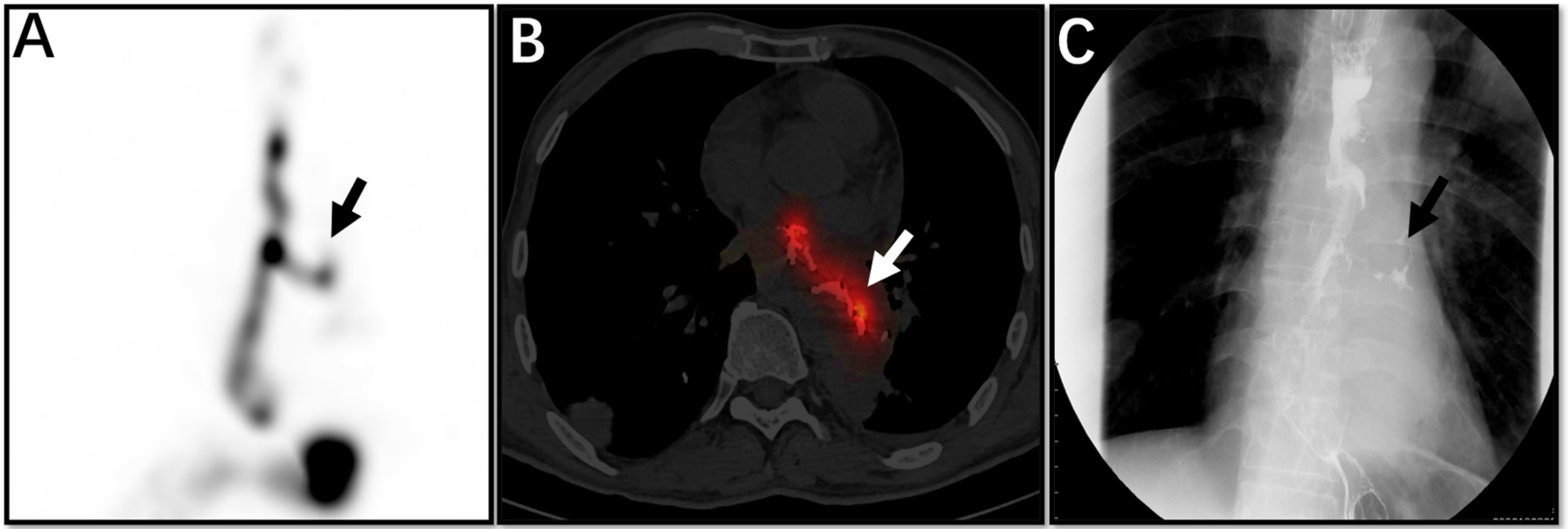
The patient was a 53-year-old man with oesophageal cancer and recurrent cough. Whole SPECT imaging (A), flued SPECT/CT imaging (B), and VFSS imaging (C) are demonstrated. These strip imaging agents are sites of oesophageal fistula (arrows). This patient underwent a barium swallow of the upper gastrointestinal tract before salivagram, so the high-density substance in the image is barium (B).
